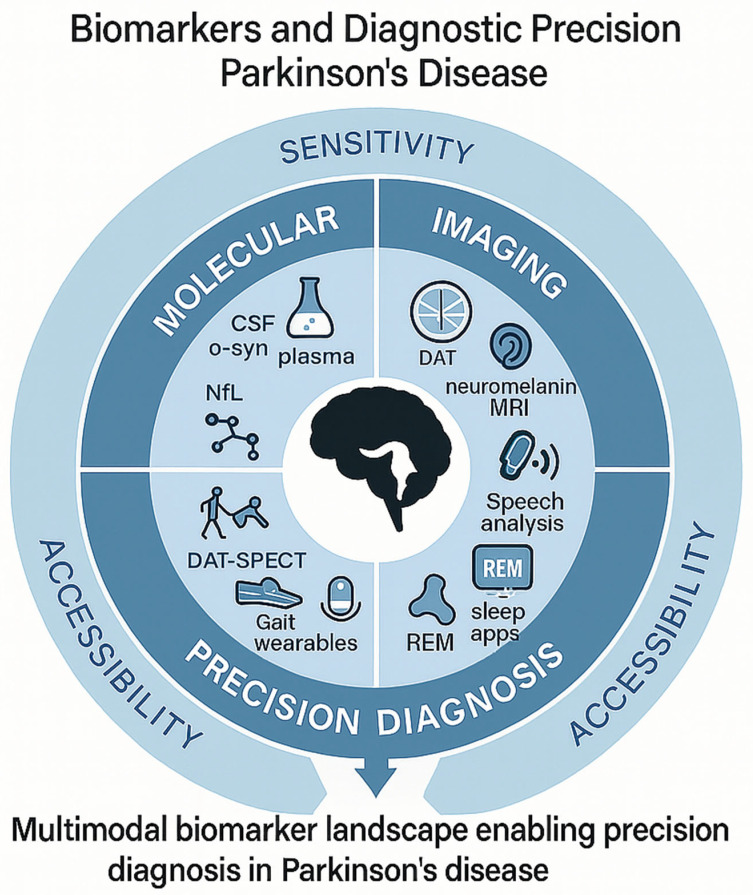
Biomarkers and Diagnostic Precision in Parkinson’s Disease (PD). Concentric rings depict three complementary domains—molecular (CSF α-syn, plasma α-syn, NfL), imaging (DAT-SPECT, neuromelanin MRI, emerging α-syn PET), and digital/clinical (wearable-derived gait metrics, speech analytics, REM-sleep apps)—arrayed around a central nigrostriatal brain silhouette. The outer band highlights key performance attributes—sensitivity, specificity, and accessibility—underscoring how integrated panels outperform single metrics and stratify patients for disease-modifying trials. α-syn, alpha-synuclein; CSF, cerebrospinal fruid; DAT, dopamine transporter; DAT-SPECT, dopamine transporter single-photon emission computed tomography; MRI, magnetic resonance imaging; NfL, neurofilament light chain PET, positron emission tomography; REM, rapid eye movement.
